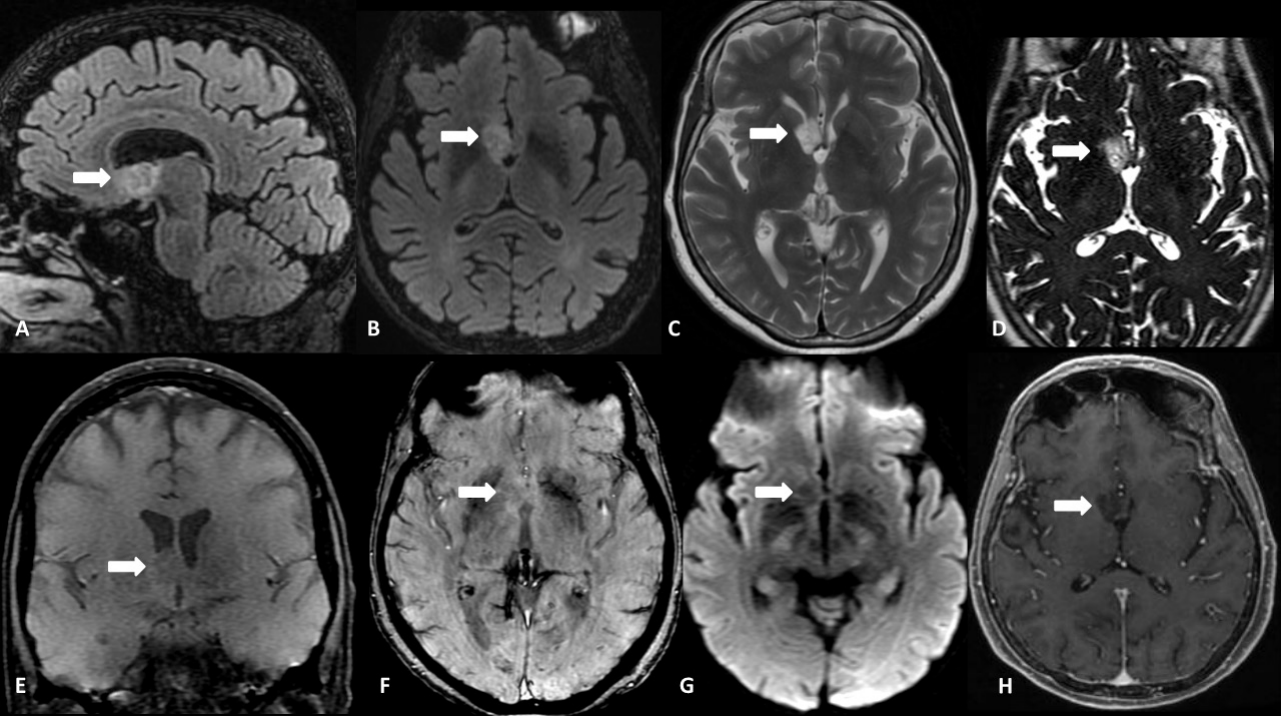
Male, 49 years old, presenting with progressive episodes of visual abnormalities and loss of consciousness. Conventional MRI show an infiltrative lesion (arrows) in the right subcallosal gyrus (septal area) and lamina terminalis, with the involvement of the chiasmatic and infundibular recesses, ipsilateral nucleus accumbens, and anterior commissure. Sagittal (A) and Axial (B) FLAIR demonstrate a bright rim with a partially suppressed central area, and Axial T2WI (C) shows a prominent hyperintense signal. Axial 3D-CISS (D) depicts a high inhomogeneous signal, confirming that the lesion is not cystic. There is a hypointense signal on both T1WI (E) and SWI (F) and facilitated diffusion on DWI (G). No abnormal enhancement is seen on Axial CE-T1WI (H).
